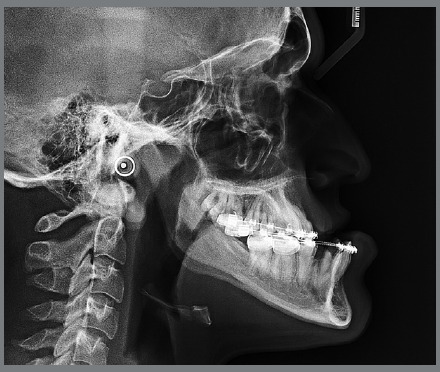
Intermediate lateral radiograph.
